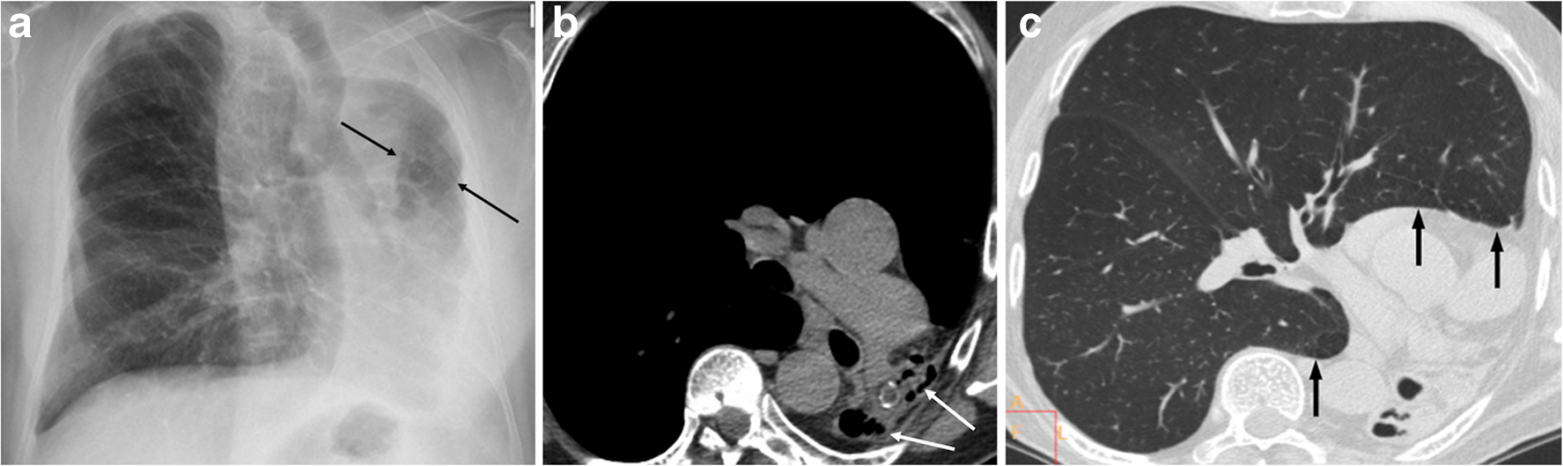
A 58-year-old male with a history of pulmonary TB 20 years previously presented with dyspnoea on exertion. (a) The chest radiograph shows marked loss of left lung volume, with an isolated hyperlucent lesion (arrows) that reflects herniation of the contralateral lung toward the left hemithorax. (b) Unenhanced multislice CT depicts total destruction of the left lung with residual cystic bronchiectasis (arrows) and herniation of the right lung toward the left hemithorax. (c) Unenhanced multislice CT clearly demonstrates herniation of the right lung toward the left hemithorax, that is, anterior herniation of the upper lobe and posterior herniation of the lower lobe (arrows)
